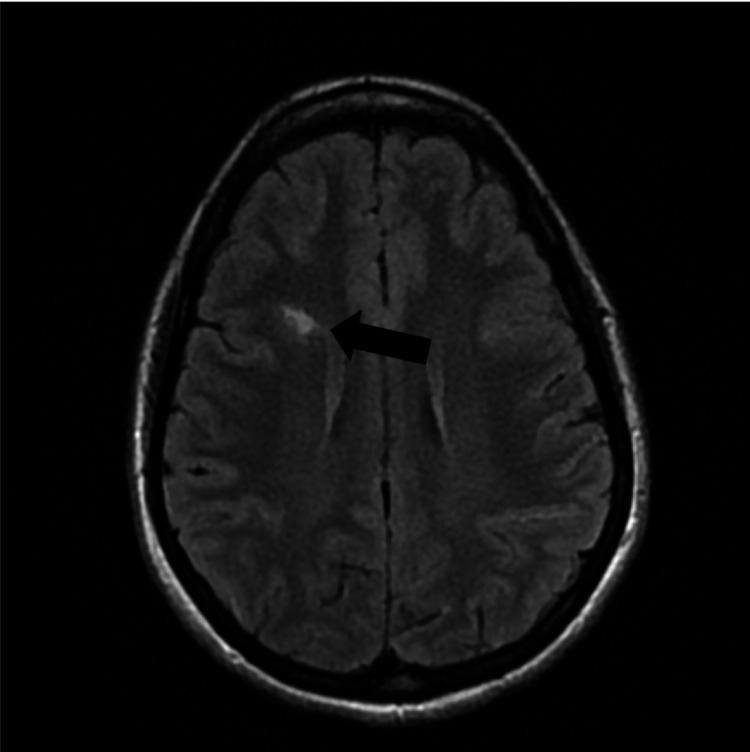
Brain MRI demonstrating a 1 cm right frontal subcortical bright T2/FLAIR hyperintensity without evidence of abnormal enhancement (black arrow).
